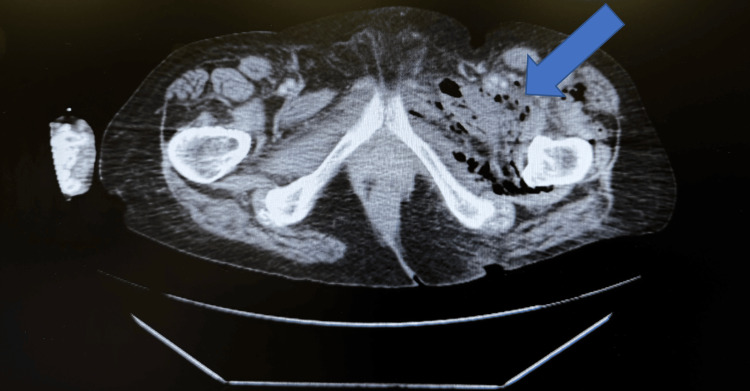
Axial CT showing extensive retroperitoneal gas extending into the pelvis, suggestive of soft tissue infection or gastrointestinal perforation with fascial plane tracking The blue arrow highlights retroperitoneal gas.
